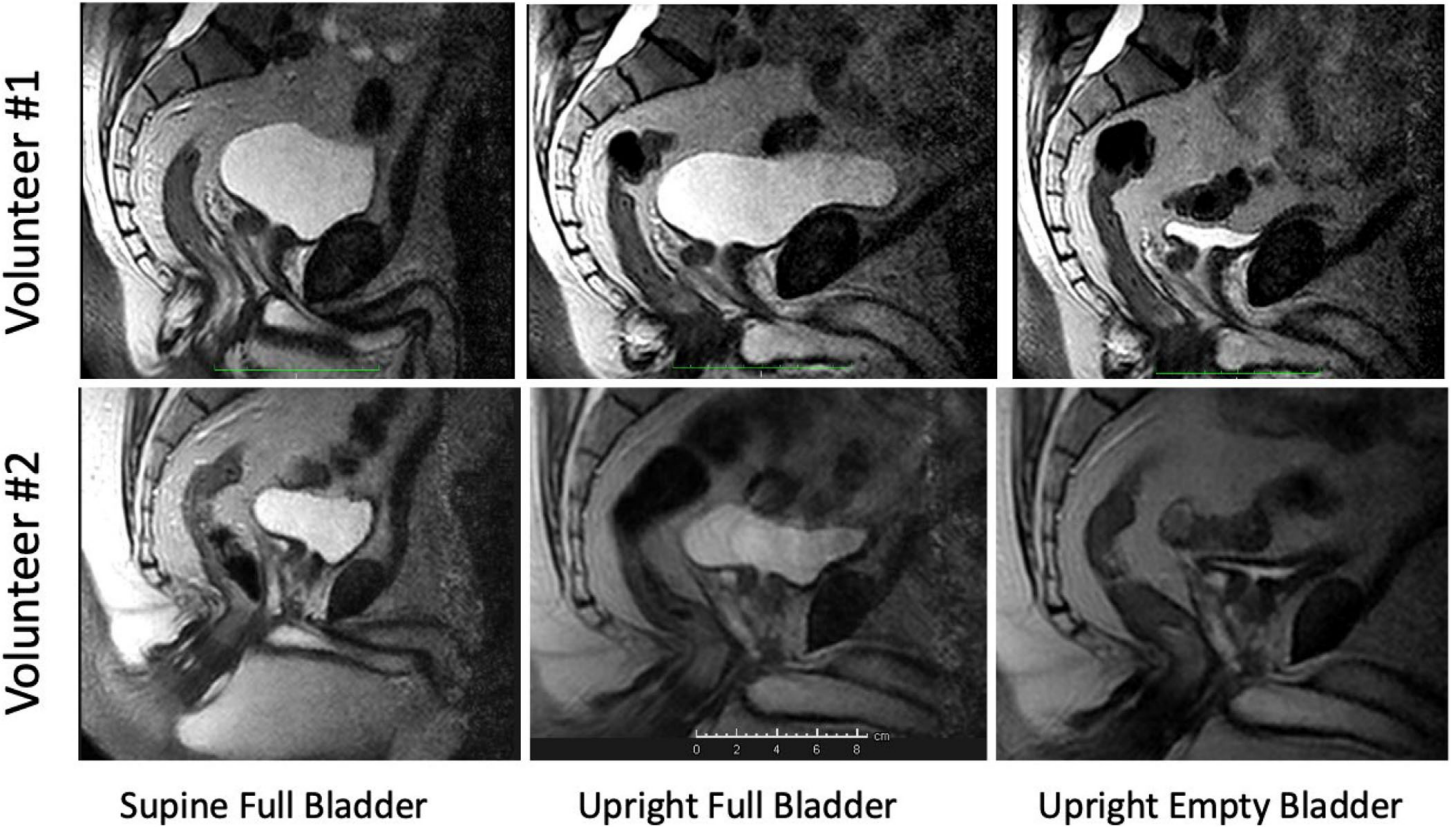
T2‐weighted sagittal MRI images in the pelvic region of Volunteer 1 (top row) and Volunteer 2 (bottom row) in each of the three conditions.
